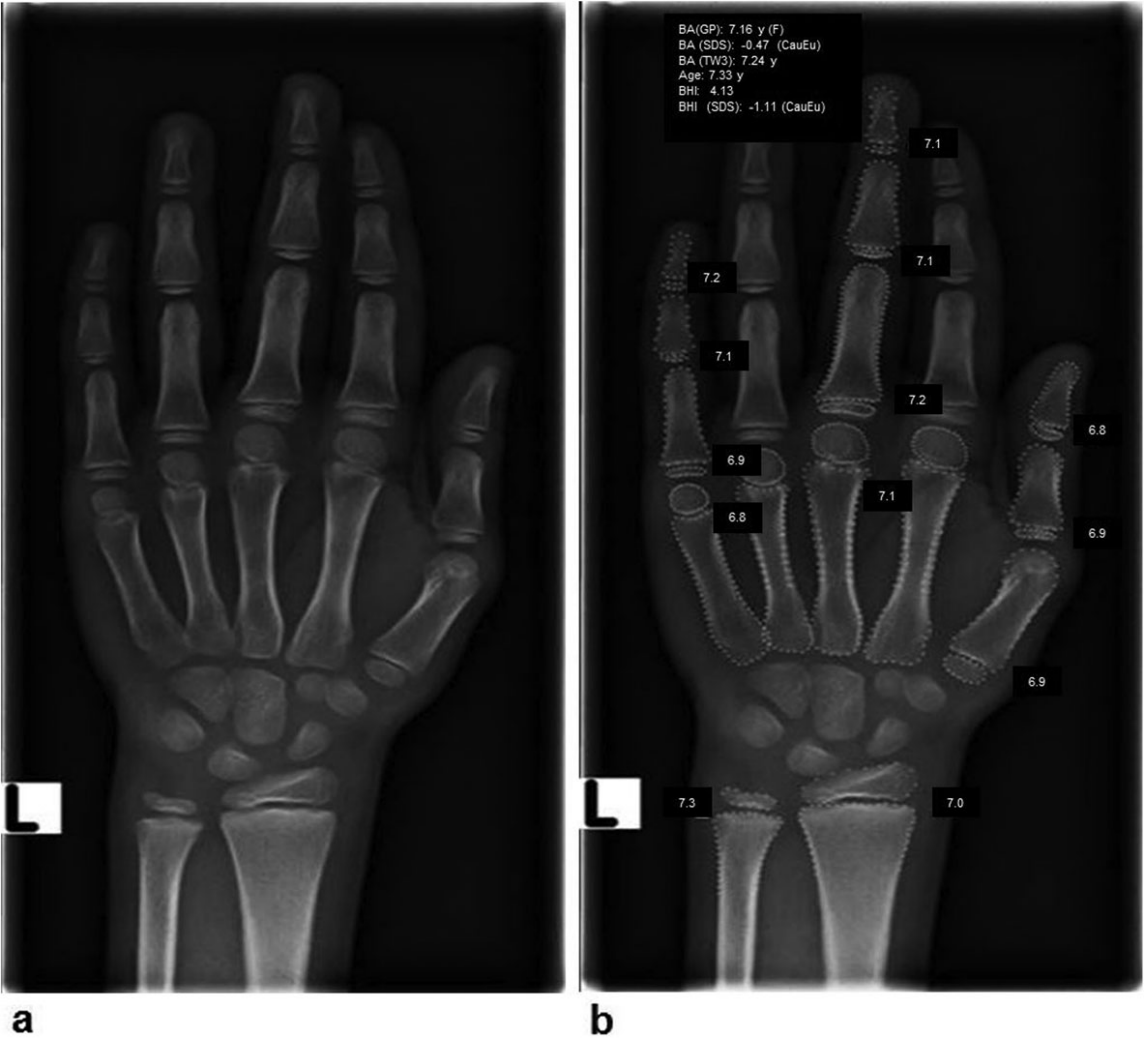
Radiograph of the left hand and wrist before (a) and after (b) automated bone age (BA) assessment by the software BoneXpert version 2.1. Bone borders of the analysed bones are automatically marked by the software. The software first calculates the intrinsic overall BA and the BA of each bone. Afterwards, these intrinsic values are transformed into Greulich-Pyle and Tanner-Whitehouse values (version TW3). The values are displayed in the annotated DICOM (Digital Imaging and Communications in Medicine) image as transformed Greulich-Pyle bone age values (BA [GP]) and Tanner-Whitehouse values (BA [TW3]) with respect to sex (F, female). Furthermore, the bone health index (BHI) is also shown with corresponding values. In addition, the standard deviation score (SDS, also known as *z*-score) is displayed concerning the corresponding population (CauEu, Caucasian European). *BA (GP)* Greulich-Pyle bone age, *BA (SDS)* Bone age standard-deviation score, *BA (TW3)* Tanner-Whitehouse bone age, *BHI* Bone health index, *BHI (SDS)* Bone health index standard-deviation score, *CauEu* Caucasian European, *F* Female
